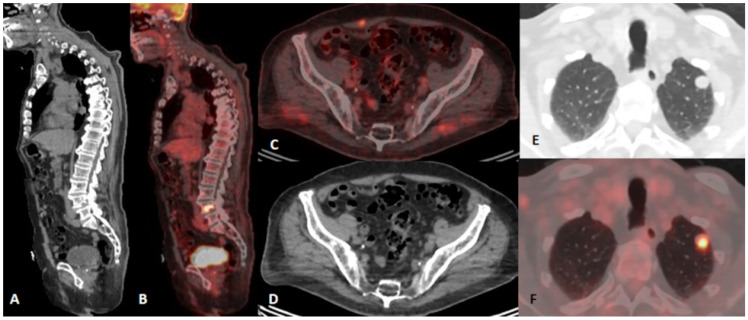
An 85-year-old male with left supraclavicular melanoma lymphonodal metastases of unknown origin. 18F-FDG PET/CT was performed for staging purposes after a possible metastatic lesion in the left lung was discovered on CT exam. 18F-FDG PET/CT indicative of metastatic involvement of the left lung and fourth lumbar vertebra and with an intramuscular lesion in the m. rectus abdominis. CT (A) and fused PET/CT (B) on the sagittal plane revealing metastasis in VL4; fused PET/CT (C) and CT (D) on the axial plane indicating metastasis in the abdominal wall. CT (E) and fused PET/CT (F) on the axial plane with an FDG avid metastatic lesion in the left lung.
